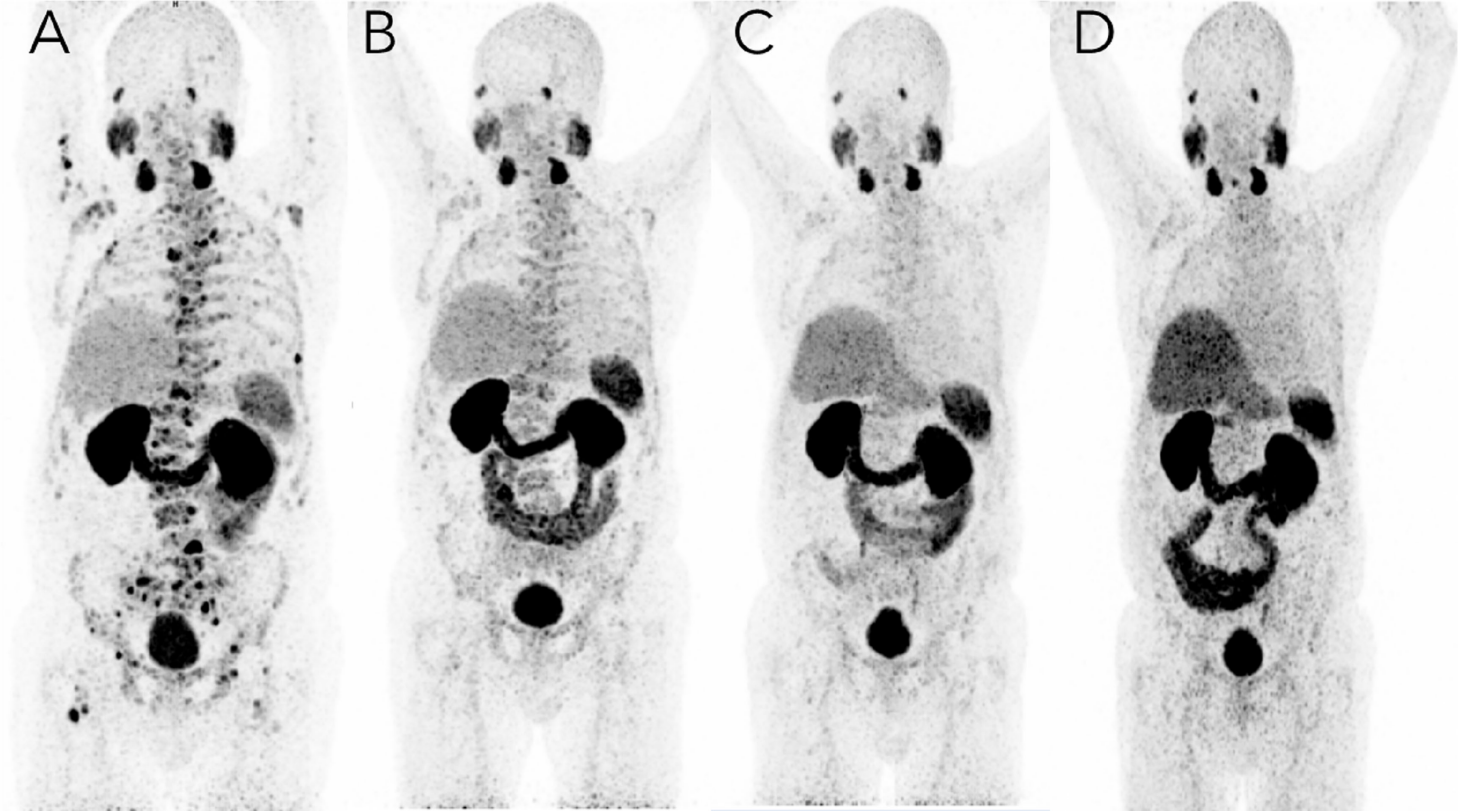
PSMA imaging before and after targeted radioligand therapy with ^177^Lu–PSMA-617. A 73-year-old male with mCRPC in 2017 with multiple bone and lymph node metastases with increased PSMA expression. (A) Imaging on March 14, 2017, just before the first dose of [^177^Lu]–PSMA-617 therapy; the baseline coronal PET [^68^Ga]-PSMA-11 PET maximum intensity projection (MIP) image shows bone metastases with significantly higher PSMA expression as well as the normal biodistribution of the tracer. (B) Imaging on July 24, 2017, just before the third dose of Lu-177 PSMA radioligand therapy, showed intense but decreasing uptake with an excellent target-to-background ratio, corresponding with molecular response to RLT therapy. Imaging four months (May 15, 2018) (C) and just over one year (February 11, 2019) (D) after the fourth dose of [^177^Lu]–PSMA-617, the [^68^Ga]-PSMA-11 PET MIP image shows multiple decreased or disappeared metastases. No evidence of PSMA-positive lymph nodes or new metastases and very mild uptake was noted in the central skeleton and sternum. Overall, no new and no intense PSMA-avid lesions were noted.
